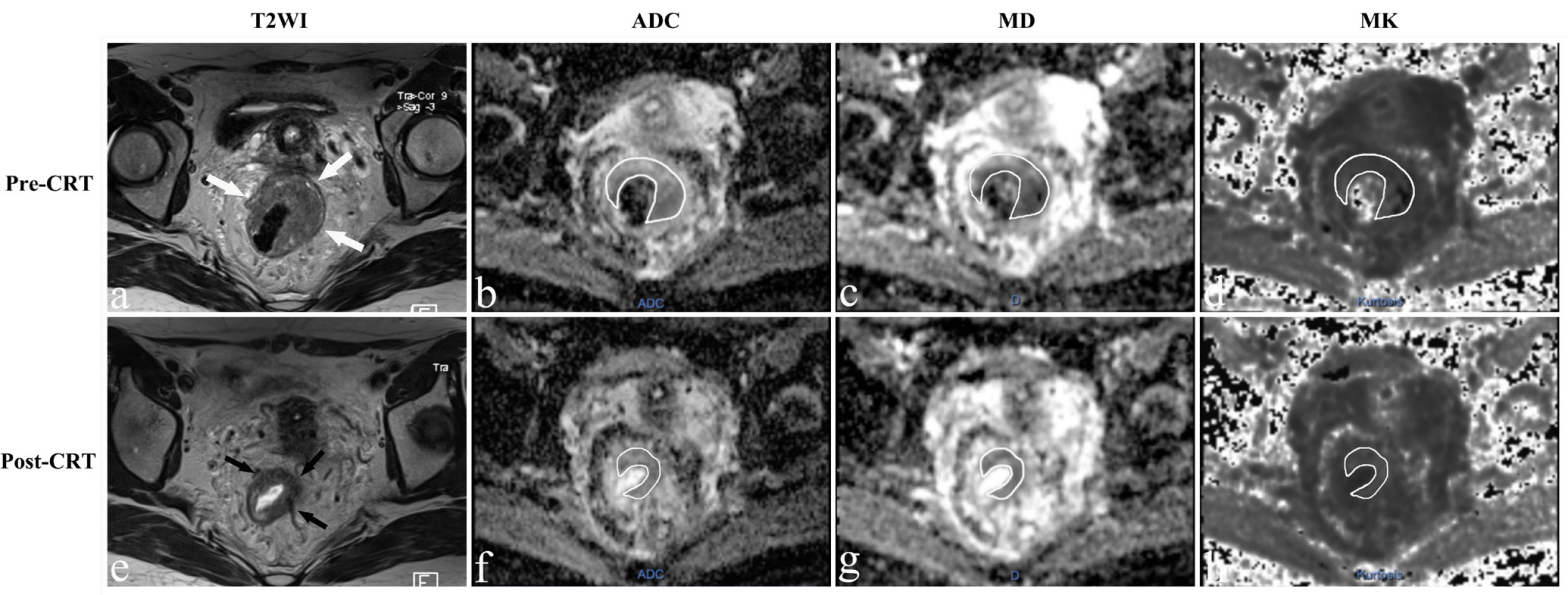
MR images of a 43-year-old woman with a non-pCR a.-e. T2-weighted MR image before and after CRT shows that there is a visible tumor with a horseshoe shape in the rectum (arrows). The ADC, MD and MK values were 0.924×10^-3^ mm^2^/s, 1.424×10^-3^ mm^2^/s, and 0.876 before treatment. After CRT, the ADC and MD increased slightly to 0.947×10^-3^mm^2^/s and 1.533×10^-3^mm^2^/s, respectively, and the MK value decreased slightly to 0.812.
